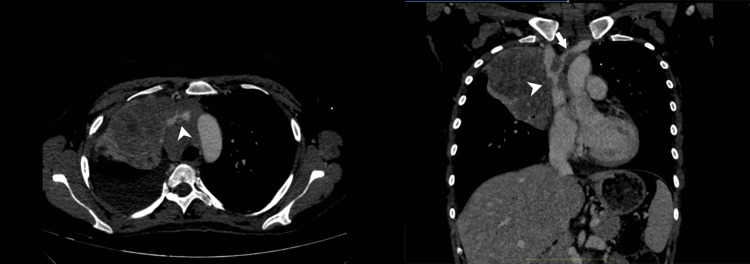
CT axial and coronal images of a patient with diffuse large B-cell lymphoma causing severe mass effect on SVC and right brachiocephalic vein
